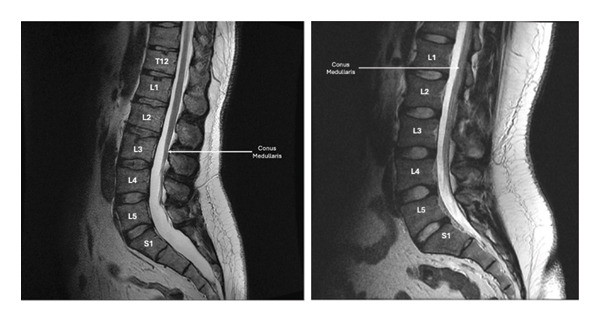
Left image: MRI showing the conus medullaris located abnormally at the L3 level in a 35‐year‐old male with tethered spinal cord syndrome (TSCS). Case courtesy of Krzysztof Nocoń, Radiopaedia.org, rID: 97956 [2]. Right Image: MRI demonstrating a normal conus medullaris position at the lower one‐third of the L1 vertebral body. Case courtesy of Bruno Di Muzio, Radiopaedia.org, rID: 43051 [3].
